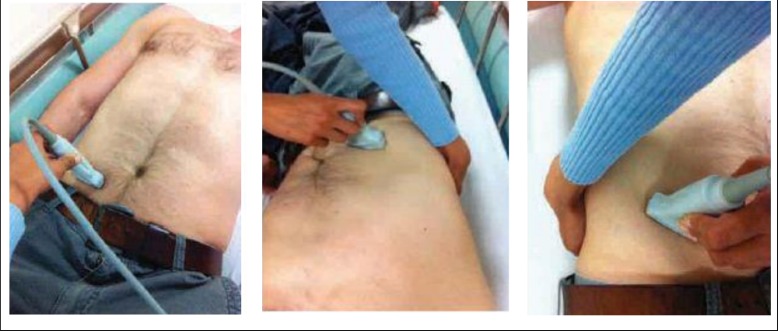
Location of probe for appendix ultrasonography.
